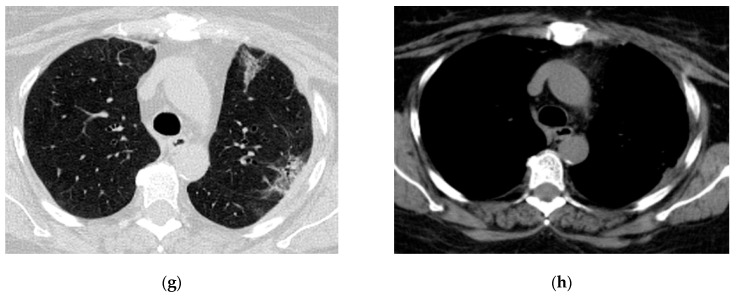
Radiologic evolution of case 2: Initial axial and coronal chest CT scans with left lower lobe abscess prior to rupture into the pleural cavity, extended bilateral lung infiltrates, and consolidations (a,b). Inserted percutaneous ICD used for both pleural lavage and drainage, trapped lung with small fluid retention (a), diffuse pulmonary infiltrates in both lung fields (c,d), and small left pulmonary cavitary lesions (d). Chest CT scans follow-up reveals intracavitary Pezzer catheter (used instead of Foley catheter due to a large soft tissue defect secondary to chest tube infection) and left-sided pyopneumothorax with mixed density fluid after lavage, ground-glass opacities (GGO) and left pulmonary consolidations areas (e,f). Six months follow-up shows complete closure of pleural and pulmonary cavities, and resolution of pulmonary infiltrates except for two small fibrotic areas (g,h).
